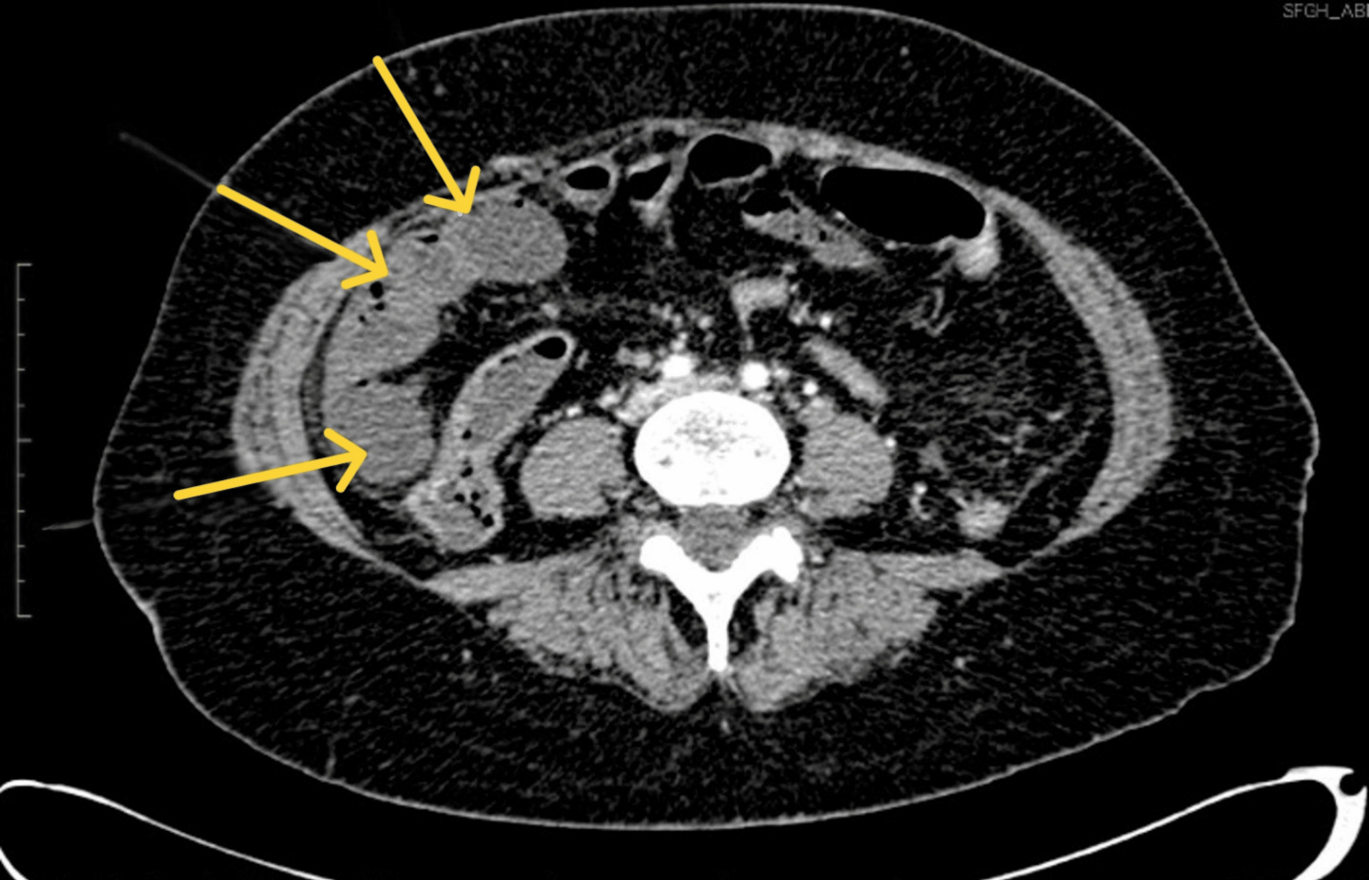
Cross-sectional CT scan of the abdomen and pelvis demonstrating nonenhancing small bowel segments (yellow arrows)
